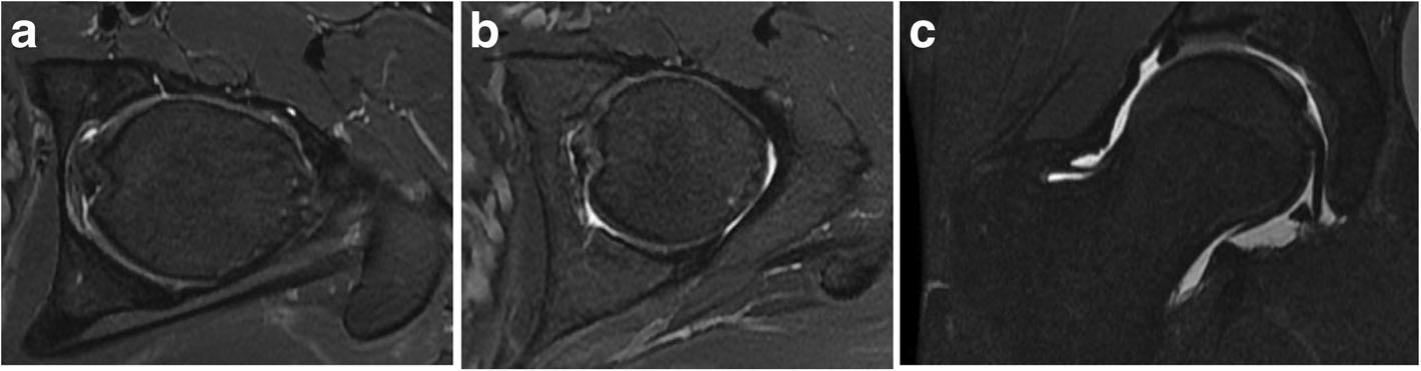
MRI findings. a Chondral rim damage. b Ligamentum teres tear. c Labral tear
